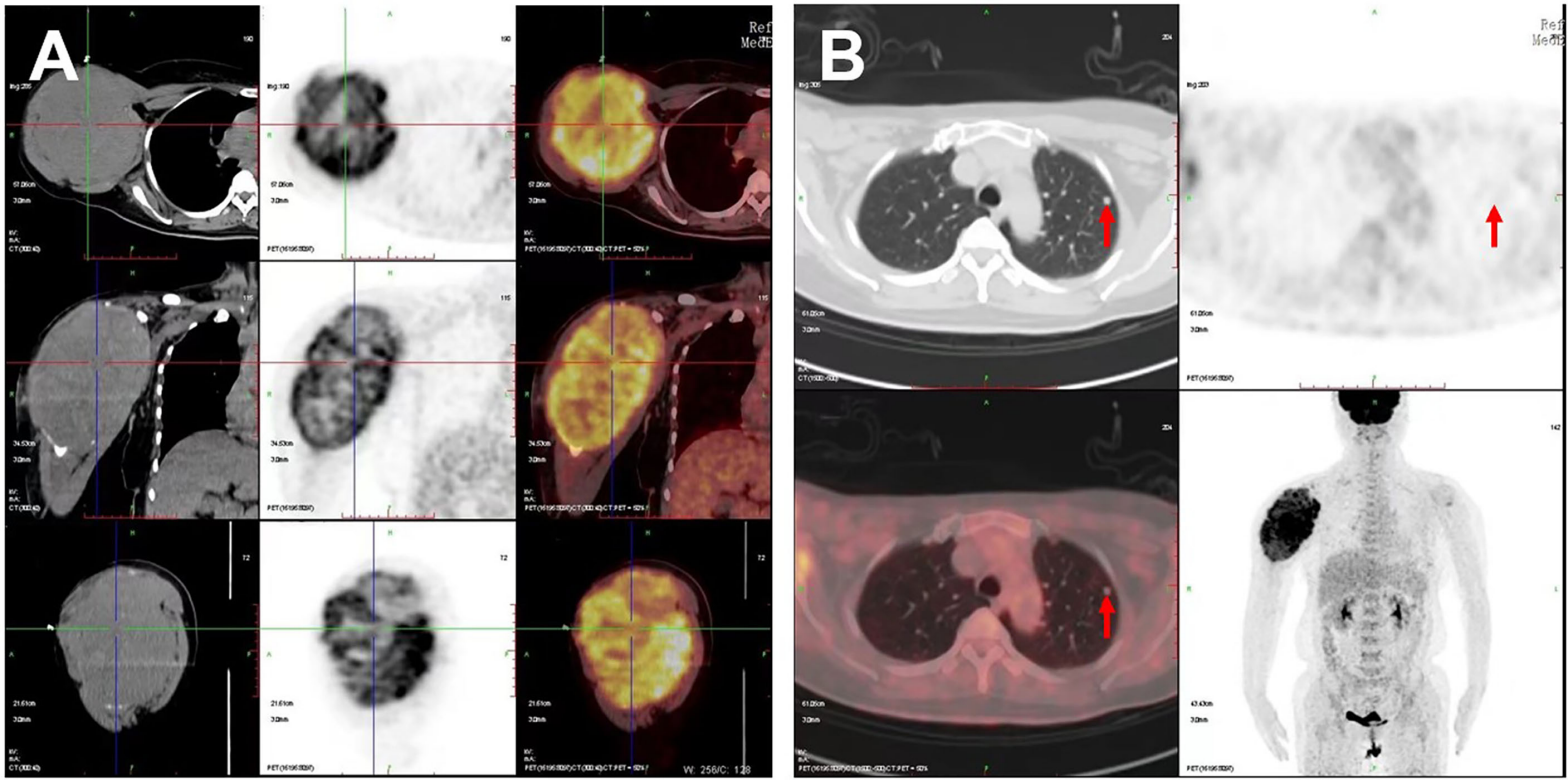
PET/CT images of the patient. Increased 18F-FDG metabolism showed in (A) the large right humerus (130 × 115 × 174 mm) on the cross, sagittal, and coronal sections and in (B) the left lung (6.5 × 6.0 mm); red arrows indicate the lung lesion.
